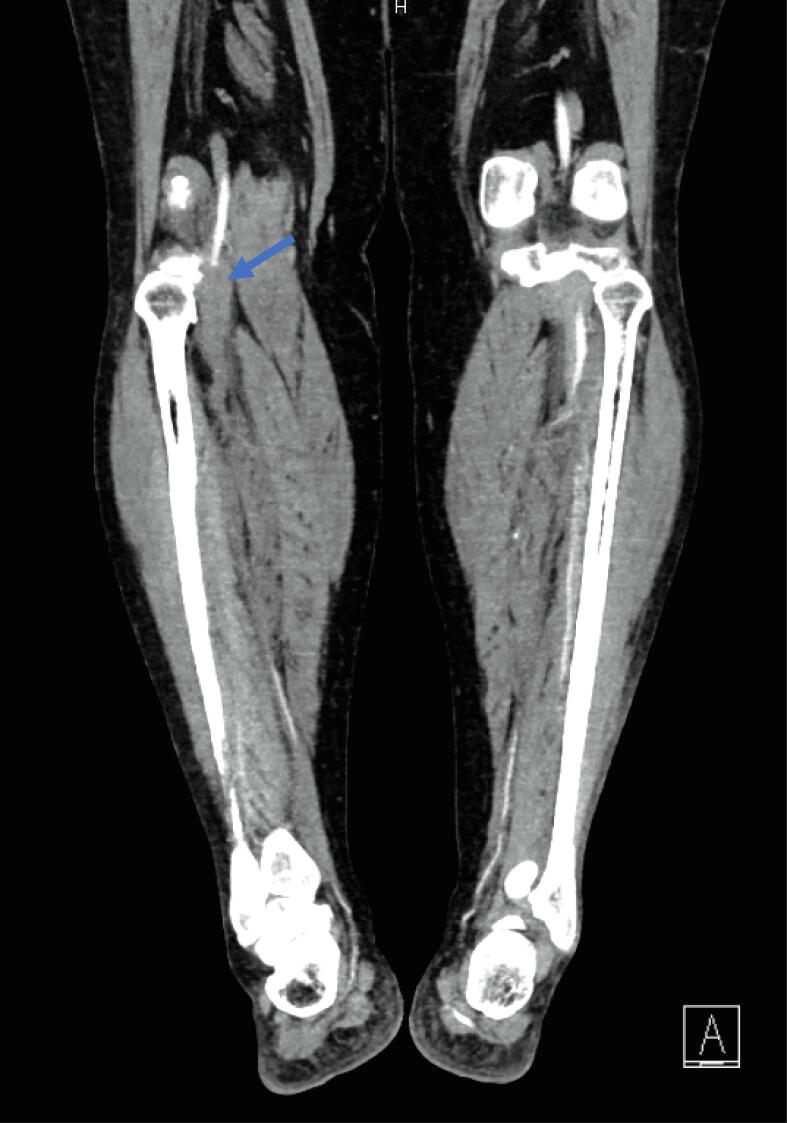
CTA images at patient presentation confirming arterial thromboembol.
